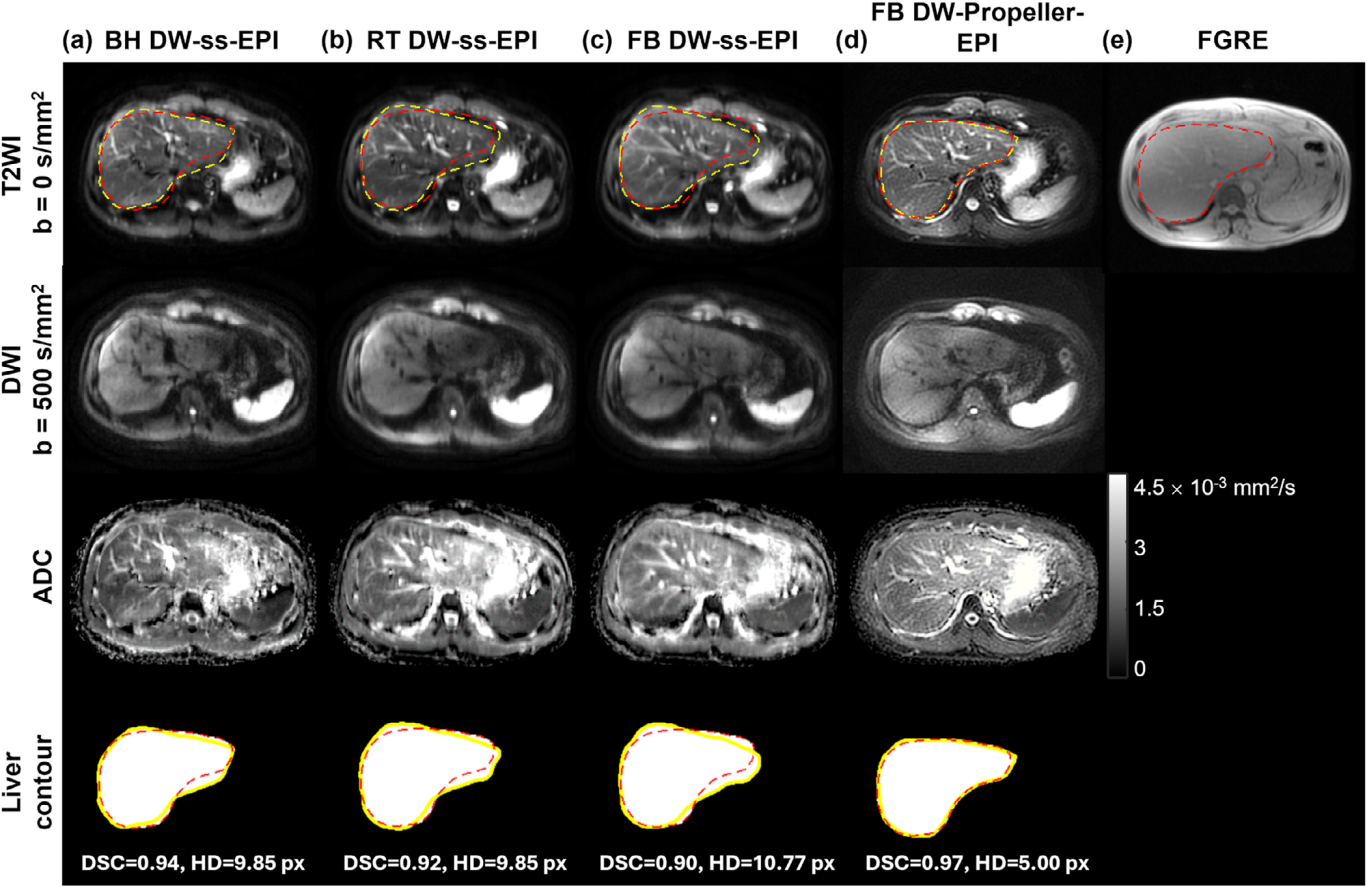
Representative liver diffusion images (at b‐values = 0 and 500 s/mm^2^) with corresponding ADC maps and obtained liver contours in a 30‐year‐old man, acquired using (a) breath‐holding diffusion‐weighted single‐shot echo‐planar imaging (BH DW‐ss‐EPI), (b) respiratory‐triggering diffusion‐weighted single‐shot echo‐planar imaging (RT DW‐ss‐EPI), (c) free‐breathing diffusion‐weighted single‐shot echo‐planar imaging (FB DW‐ss‐EPI), and (d) free‐breathing diffusion‐weighted Propeller echo‐planar imaging (FB DW‐Propeller‐EPI). The yellow dashed lines represent the liver contours from four techniques, and the red dashed lines indicate the gold‐standard liver contour obtained from (e) fast gradient echo (FGRE) image overlaid after centroid‐based registration. Dice Similarity Coefficient (DSC) and Hausdorff's Distance (HD) between the contours obtained from each technique and the FGRE image were calculated to quantitatively evaluate contour agreements. DWI images and ADC map obtained with FB DW‐Propeller‐EPI show superior image quality and geometric fidelity compared to the images obtained with the three DW‐ss‐EPI techniques. The liver contour from FB DW‐Propeller‐EPI had the largest DSC (0.97) and the smallest HD (5.00 px) values. The DWI image (on the second row) from FB DW‐Propeller‐EPI received higher ratings than the other 3 techniques across all five image quality aspects. Geometric fidelity scores (average of two raters) for BH DW‐ss‐EPI, RT DW‐ss‐EPI, FB DW‐ss‐EPI, and FB DW‐Propeller‐EPI techniques were: 3.5, 4, 3, and 5, respectively.
